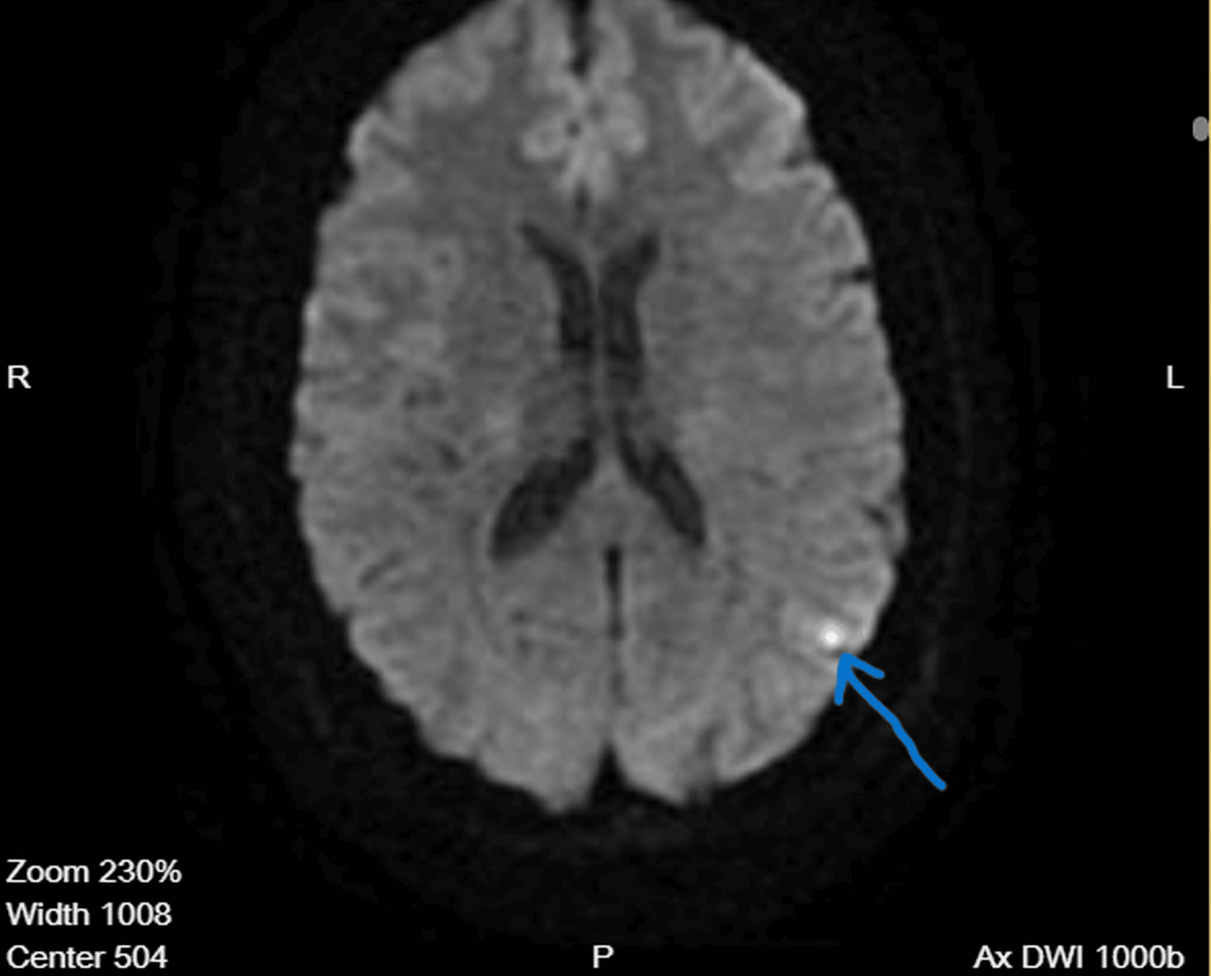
Diffusion-weighted MRI of the brain Diffusion-weighted magnetic resonance imaging (DWI) demonstrates a small punctate cortical focus of restricted diffusion in the left parietal lobe, corresponding to the distal M4 territory of the left middle cerebral artery. The arrow indicates the area of acute ischemic infarction.
